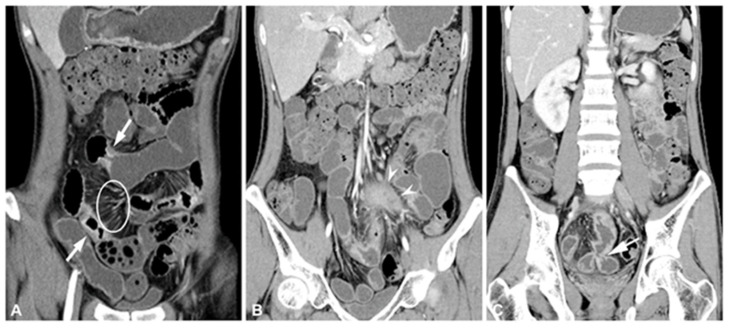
Diagnosis of inflammatory Crohn’s disease with extraenteric complications by computed tomography enterography. (A) Multifocal segmental stricture showing wall thickening, mural hyperenhancement, and stratification shown by arrows and engorged vasa recta, comb sign highlighted by a circle. (B) Mesenteric abscess is shown by arrowheads. (C) Adjacent to the enteroenteric fistula shown by the arrow. Reprinted with permission of [57].
